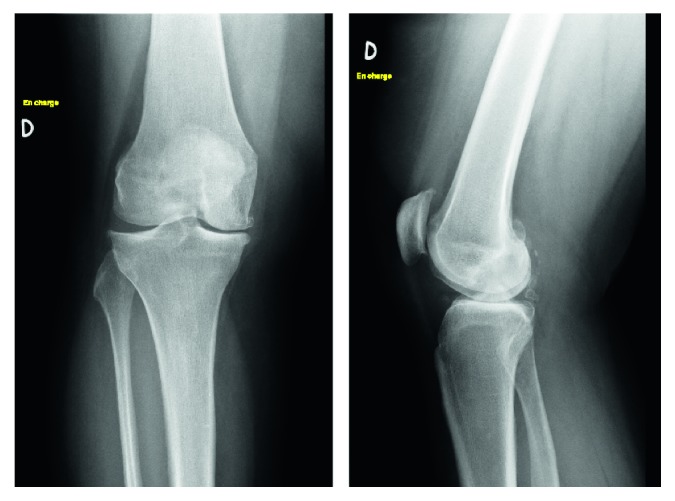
Preoperative weight-bearing X-rays showing narrowing of the joint space in the internal femorotibial compartment.
